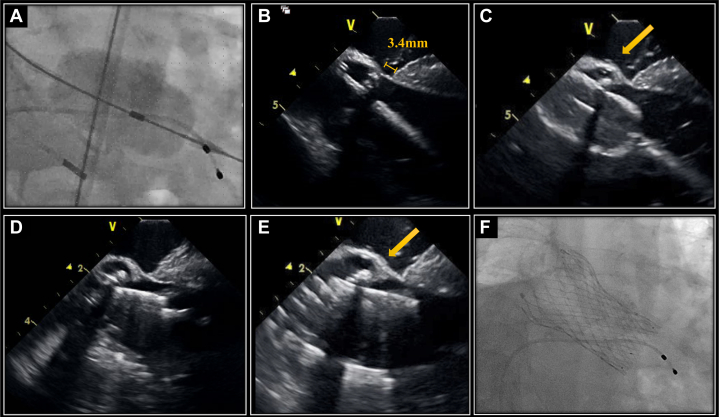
Fluoroscopic and Intracardiac Echocardiography Images During Procedures (A) Fluoroscopic view of a predilation using a 22-mm Inoue balloon. (B) Intracardiac echocardiography (ICE) image of membranous septum (MS). (C) ICE image showing calcification between the balloon and the MS during predilation. (D) ICE image at the point of no recapture. (E) ICE image showing calcification between Evolut valve and the MS after valve deployment. (F) Fluoroscopic view of a valve in the cusp overlap view.
